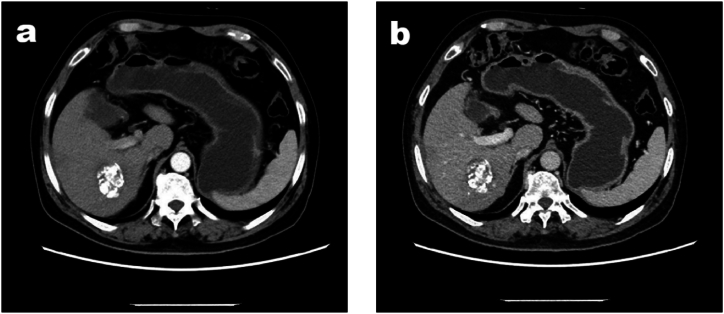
(a). Enhanced CT (arterial stage) showed that the tumor was located in the right posterior lobe, with internal iodol deposits and no enhancement. Fig. 3b. Enhanced CT (venous phase) showed that the tumor was located in the right posterior lobe, with internal iodol deposits.
